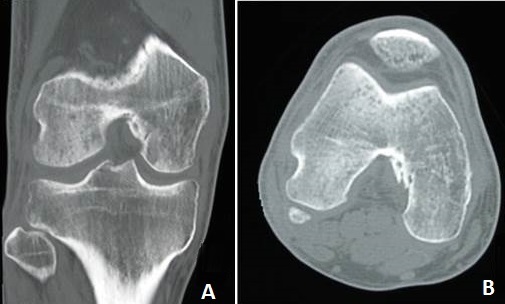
Control CT scan of right knee is highlighting a perfect consolidation of bone lesions. (A) coronal; (B) axial
